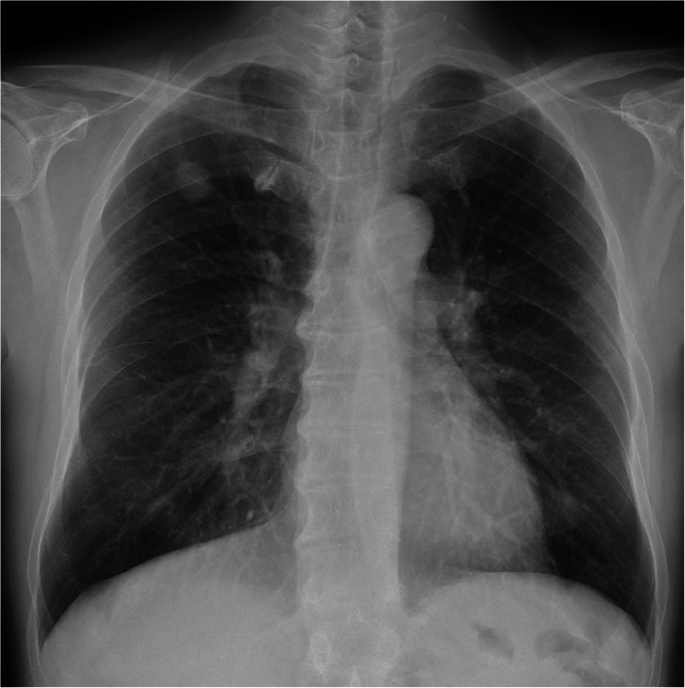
Chest roentgenogram revealing a well-defined nodule in the right upper lung field.
